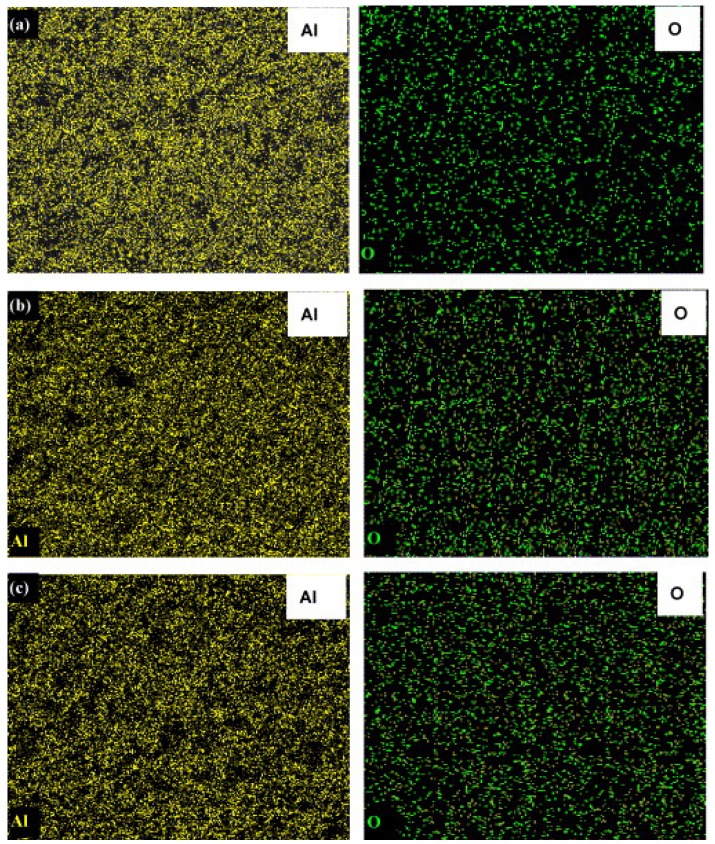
X-ray maps showing Al (left) and O (right) for the Al–Al_2_O_3_ (50 nm) powders milled for 20 h: (a) 20 vol% Al_2_O_3_; (b) 30 vol% Al_2_O_3_; and (c) 50 vol% Al_2_O_3_ [13].
